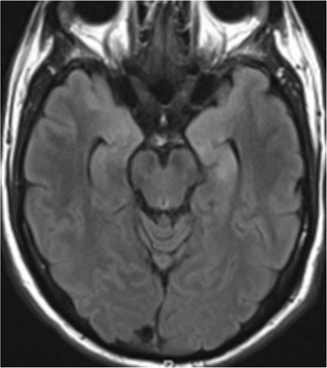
A 52-year-old male with known diagnosis of small cell lung carcinoma and new onset seizures. Increased FLAIR signal is noted in the mesial temporal lobes, left greater than right without mass effect or volume loss. Based on positive serum anti Hu antibodies and imaging findings, the diagnosis of paraneoplastic limbic encephalitis was made
